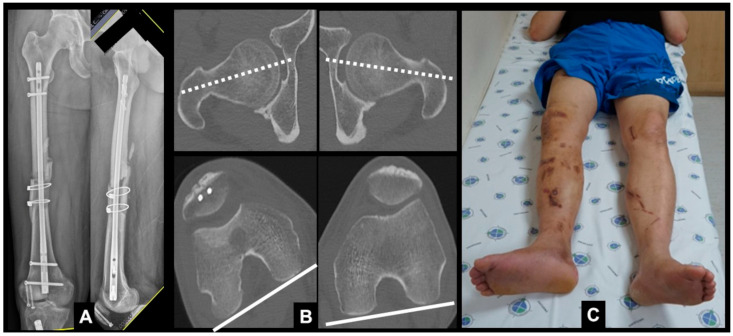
(A) Preoperative anteroposterior and lateral radiographs showing nonunion after retrograde nailing; (B) CT scan showing an evident difference in the femoral torsional angle between the affected and unaffected sides; (C) externally rotated foot on the right side indicating retroversion of the femur.
